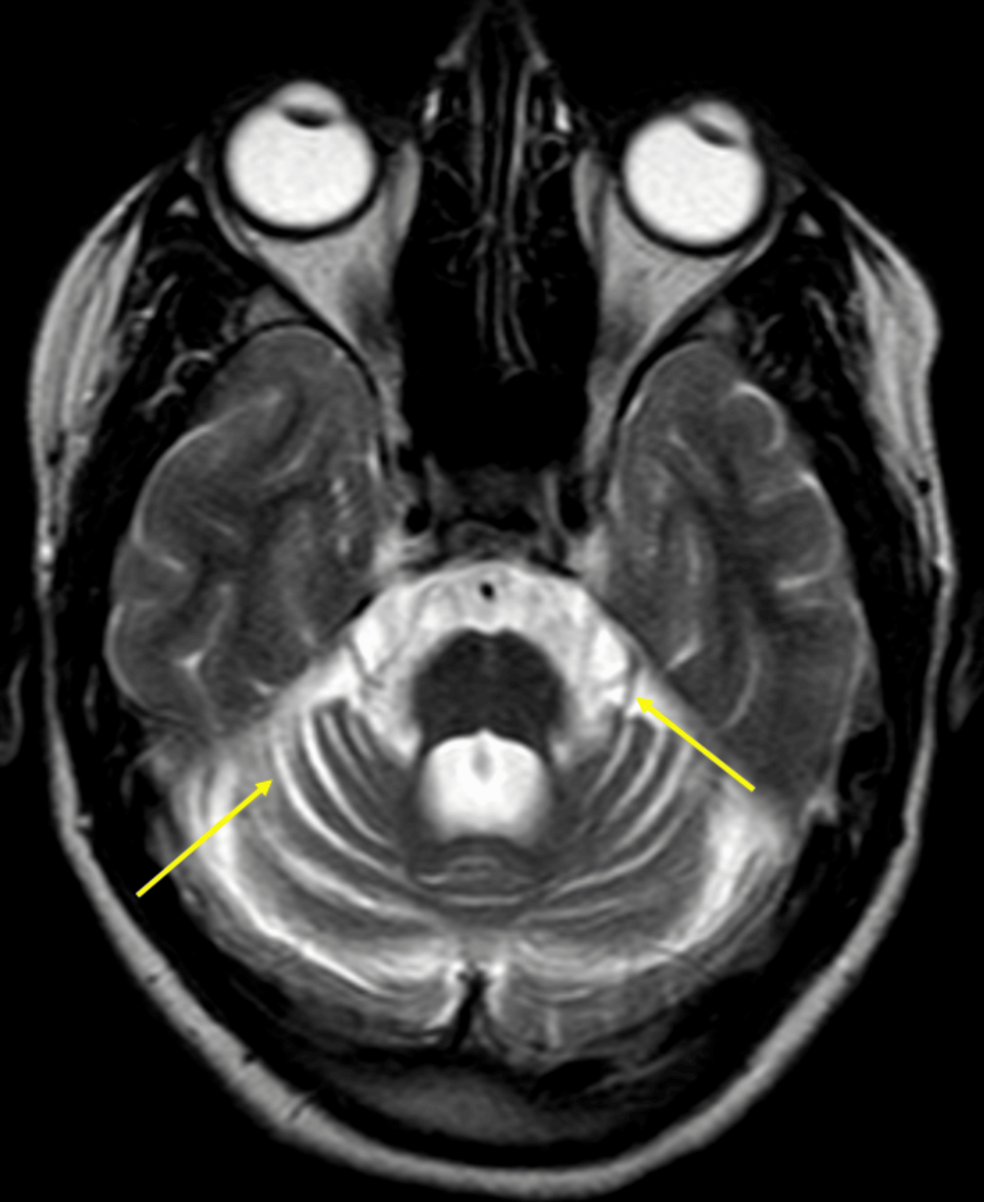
The axial T2-weighted image shows a higher signal in the cerebellar white matter and the brainstem, reflecting gliosis or degeneration. The cerebellar atrophy is again evident with dilatation of the fourth ventricle and prominent cerebellar fissures. The T2-weighted image is useful for visualizing the extent of white matter changes and atrophy.
